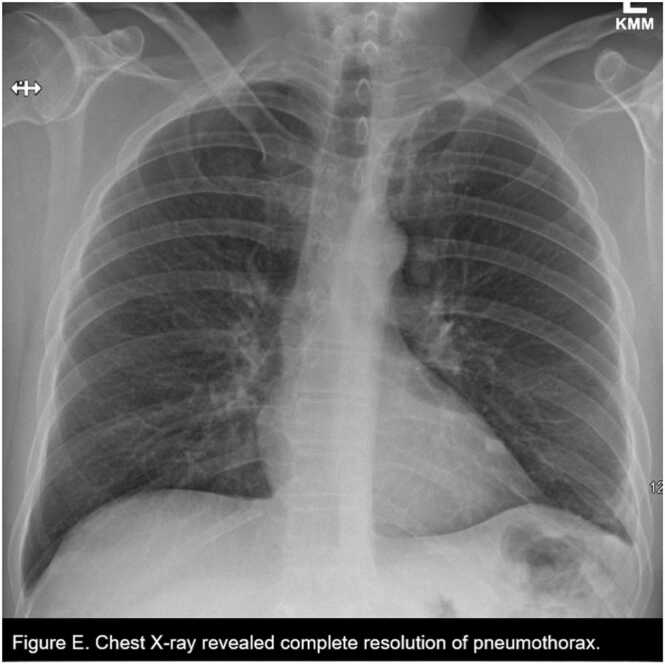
Chest X-ray revealed complete resolution of pneumothorax.
